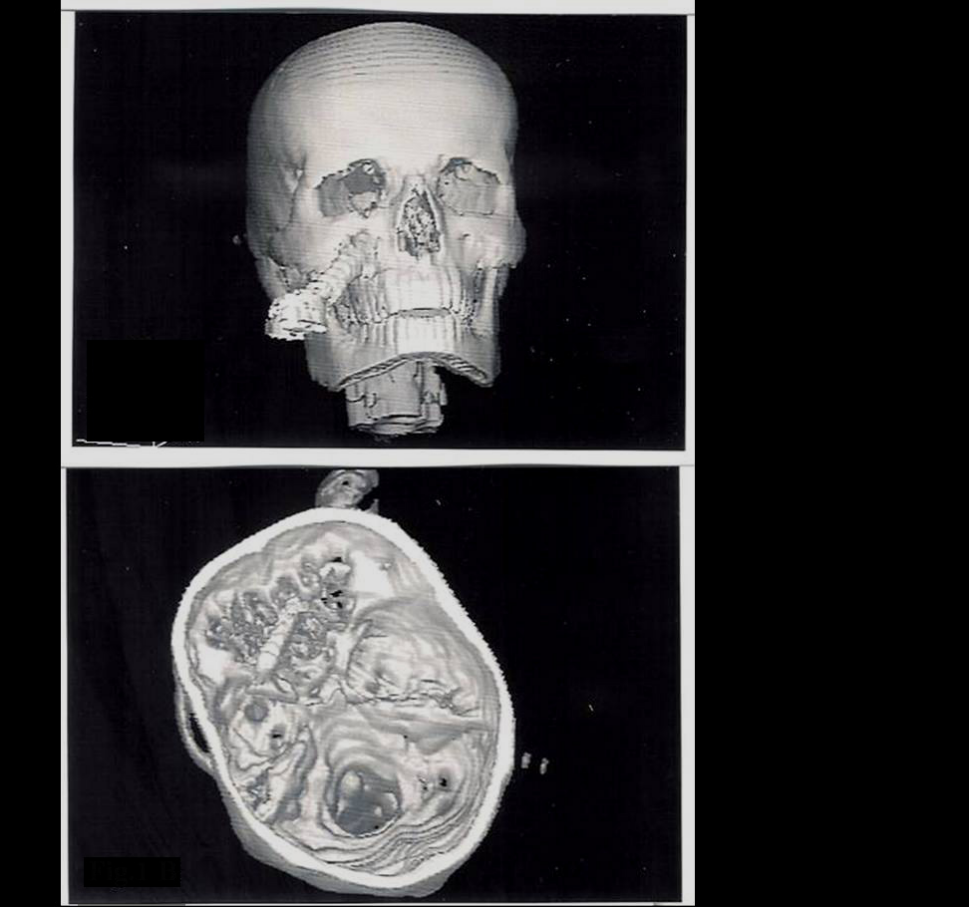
A 3-D CT scan revealed the severity of the skull base penetrating injury.
